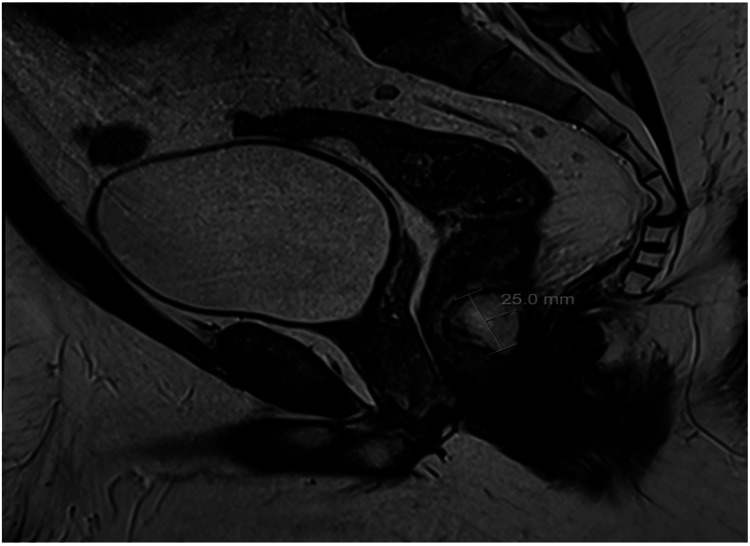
Rectal wall abscess 25 mm in diameter on T2-weighted images on MRI (sagittal view).
